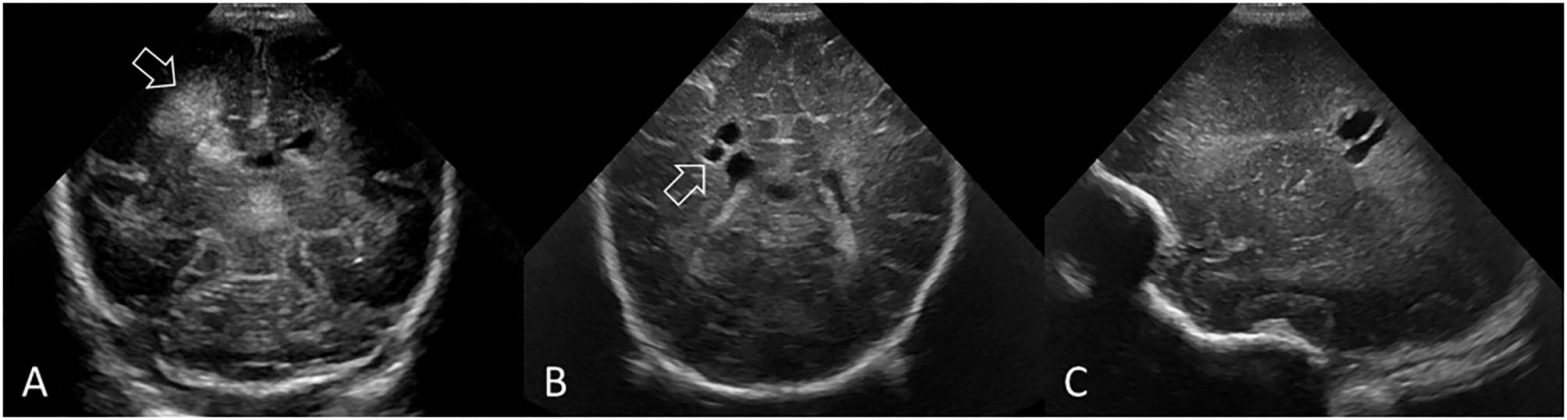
PVHI with eventual evolution into multiple, unilateral cystic lesions separate from the lateral ventricle in a preterm infant (GA 25 weeks). (A) Coronal ultrasound scan 5 days after birth showing the right-sided GMH-IVH and large echodensity in the periventricular white matter adjacent to, but not communicating with, the lateral ventricle (arrow). (B,C) Coronal and right parasagittal scans 11 weeks later show cystic lesions in the parietal white matter that do not communicate with the lateral ventricle (arrow). PVHI adjacent to, but not communicating with, the lateral ventricle often evolves into multiple smaller cystic lesions rather than one large porencephalic cyst. This small cluster of white matter cysts could be mistaken for grade 2 ischemic WMI. However, review of the baseline cUS exam and understanding the evolution of injury clarifies that this represents variant cystic evolution of PVHI.
